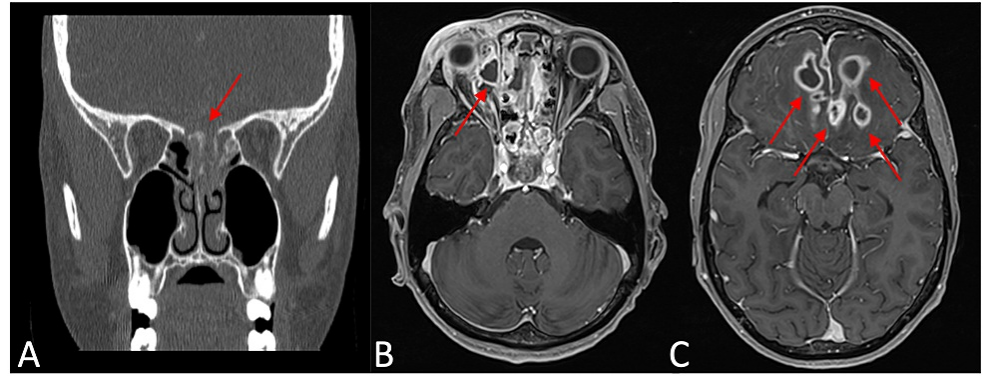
Preoperative CT and MRI images obtained after initial presentation. (A) Coronal CT showing dehiscence of the cribriform plate and opacification of left posterior ethmoid cells; (B) T1 axial MRI showing subperiosteal abscess in right medial orbit; and (C) T1 axial MRI showing bilateral frontal lobe abscesses.
